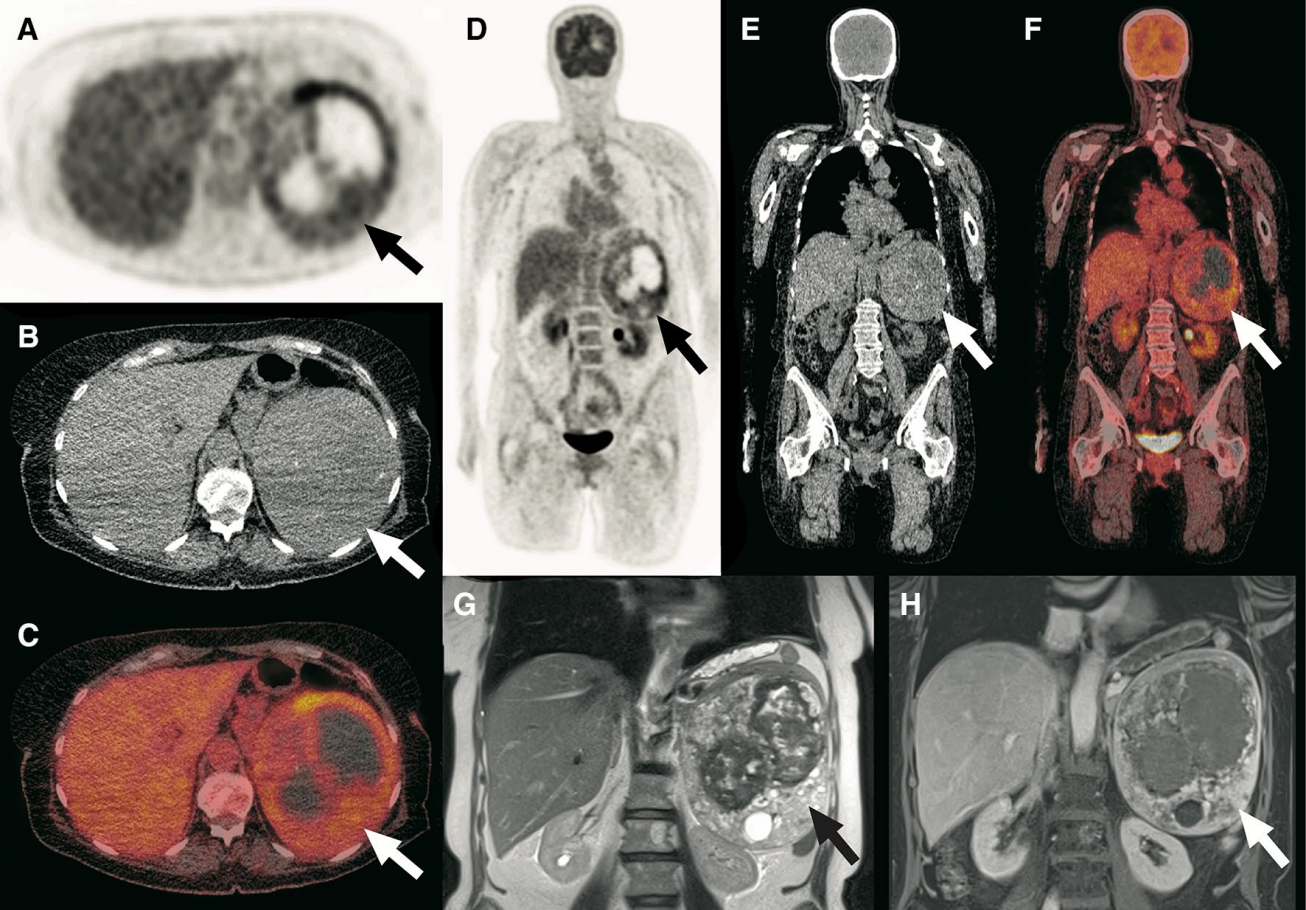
A 71-year-old woman presented to the rheumatology and immunology department because of fatigue, general malaise and involuntary weight loss of 9 kg in 5 months. She did not have fever or abdominal pain. Blood tests showed a CRP level of 256 mg/L and a white blood cell count of 11.0 × 10^9^/L. An FDG-PET/CT scan was ordered to assess the possibility of vasculitis, infection, or malignancy. Axial FDG-PET (a), low-dose CT (b), and fused FDG-PET/CT (c) showed an abnormal spleen (arrows) that was markedly enlarged with inhomogeneous areas of pathologic FDG uptake and central photopenic areas that could not be clearly assessed on the low-dose CT (b). Coronal FDG-PET (d), low-dose CT (e), and fused FDG-PET/CT (f) demonstrated similar findings (arrows). No pathologic FDG-avid foci were detected elsewhere. Based on the laboratory and FDG-PET/CT findings, splenic cyst infection was strongly considered, although other conditions (in particular malignancy) could not be excluded. MRI was ordered for further assessment of the spleen. Coronal T2-weighted (g) and gadolinium-enhanced fat-suppressed T1-weighted (h) images showed a markedly enlarged and inhomogeneous spleen (arrows) with cyst-like changes, and centrally both T2 hypointense and hyperintense non-enhancing components. The differential diagnosis based on the MRI examination included hemangioma with hemorrhage, infected splenic cyst with abscess formation, and angiosarcoma. Splenectomy was performed 1 day later, which demonstrated angiosarcoma with central hemorrhagic areas
